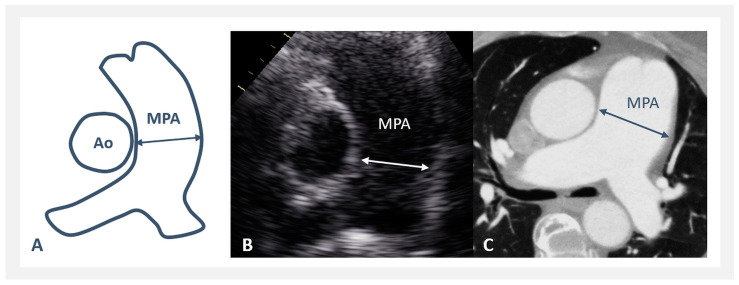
Pulmonary artery measurement: (A) scheme; (B) by echocardiography; and (C) by computer tomography (MPA—main pulmonary artery; Ao—aorta).
